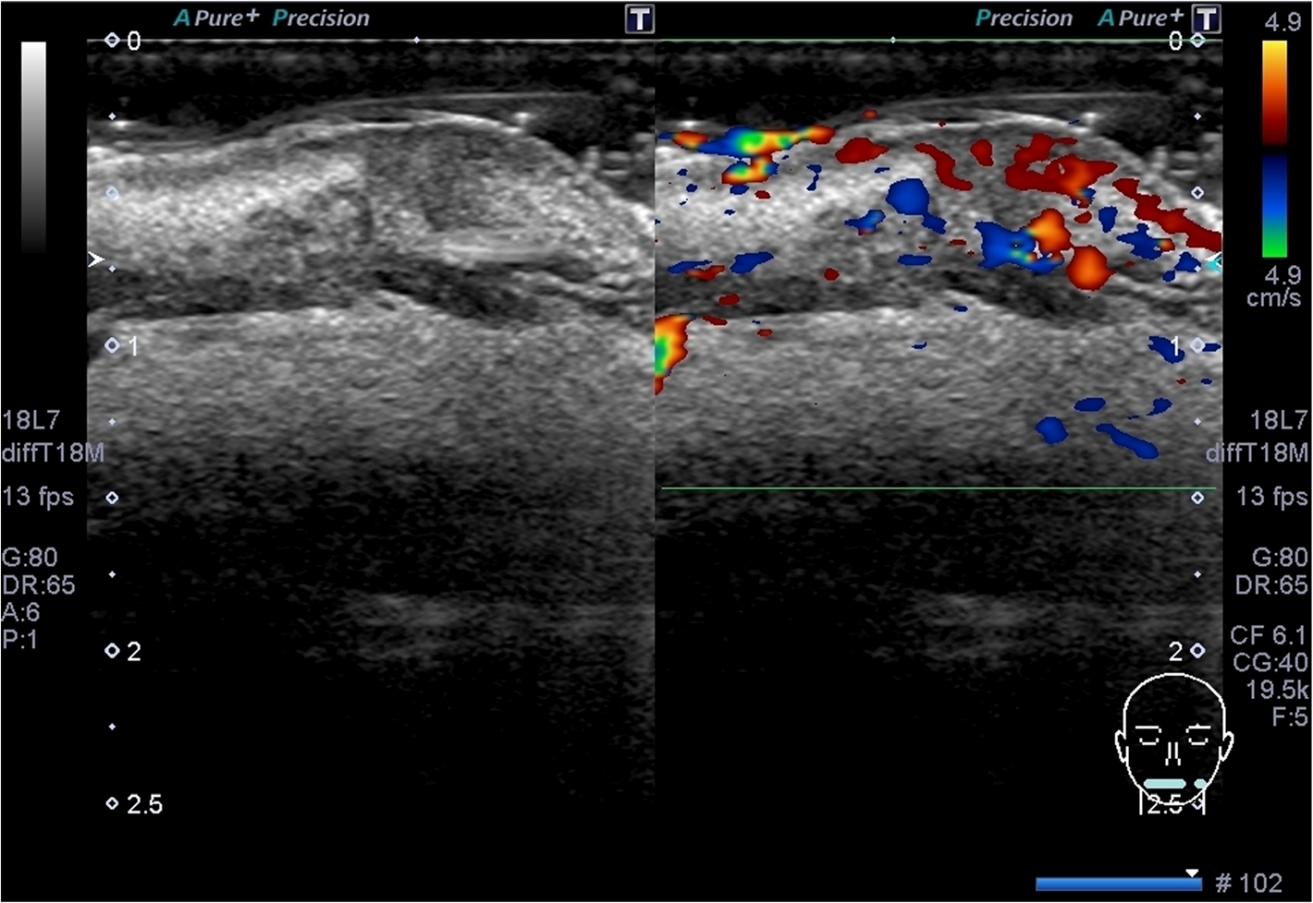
Sonograms of the lesion on the lower lip after treatment with amoxicillin. A gray-scale transverse sonogram (*left*) and color Doppler sonogram (*right*) of the lesion at 3 months after the initiation of treatment. Her lip lesion showed remarkable improvement at 3 months after treatment initiation, with only a hypoechoic lesion with blood flow signals remaining
